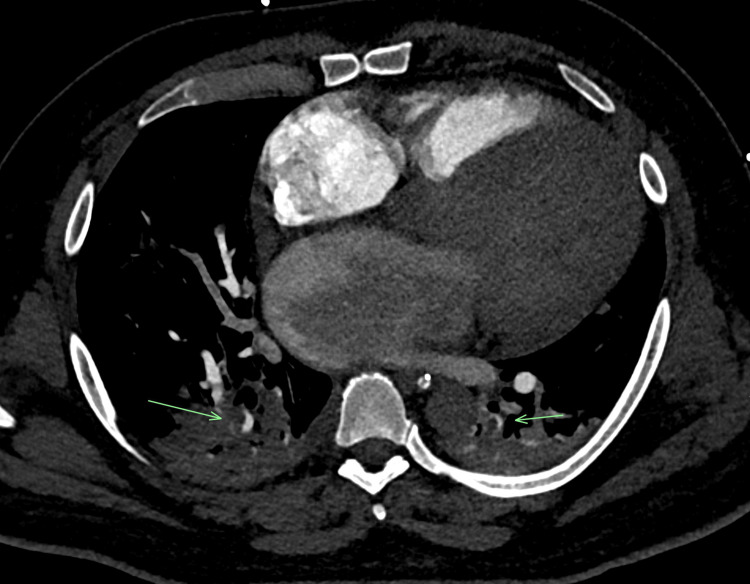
Computed tomography angiography (CTA) of the chest showing bilateral filling defects in the lungs demonstrative of bilateral pulmonary emboli
